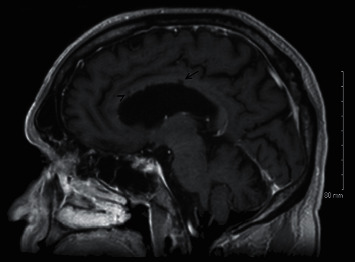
Sagittal T1-weighted slice at the level of the corpus callosum 12 months after treatment initiation. *Note*. The decreased “snowball” lesion in the body of the corpus callosum (demarcated by arrow) and relative persistence of linear “spoke” or “icicle” lesions (demarcated by an arrow head).
